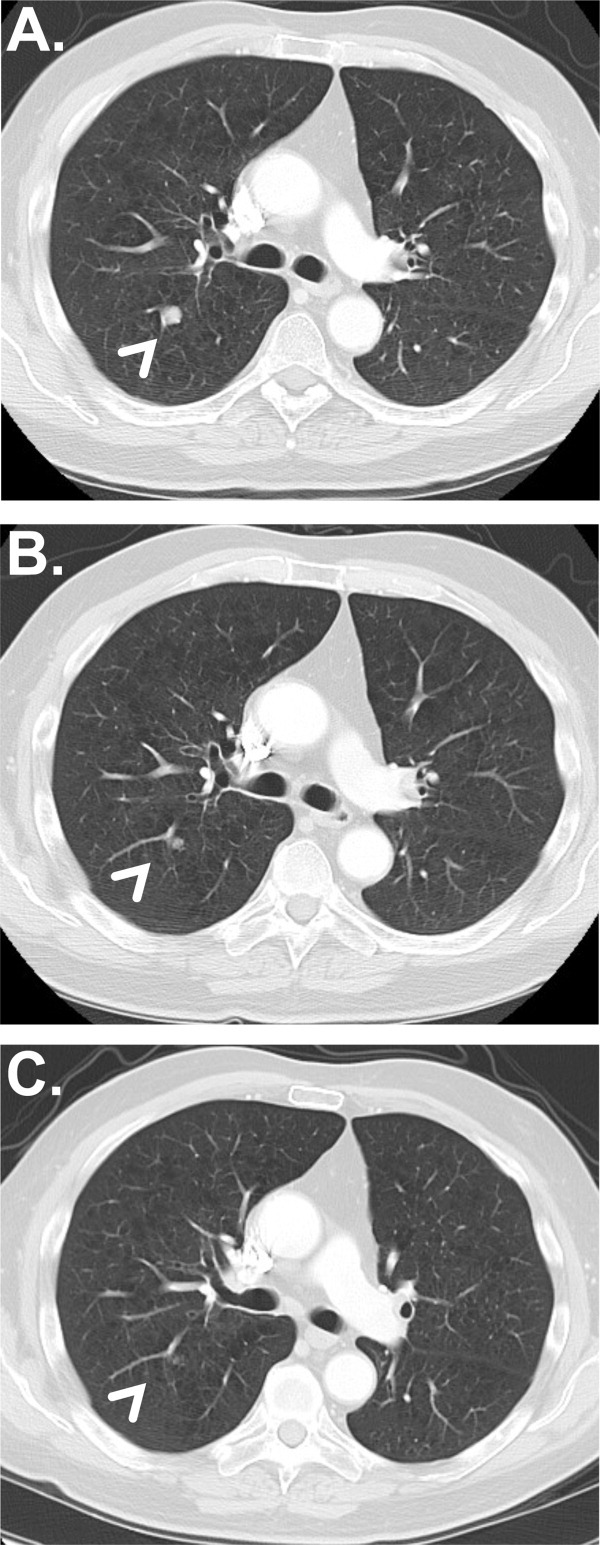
Radiographic images of a patient with a complete response are shown. Representative images were obtained (A) at baseline, (B) after completing induction with 4 treatments of ipilimumab every 3 weeks, and (C) at 9 months (before the second dose of maintenance ipilimumab). The patient was alive at the time of last follow-up without evidence of disease recurrence, 11.2 months after the initiation of protocol therapy.
